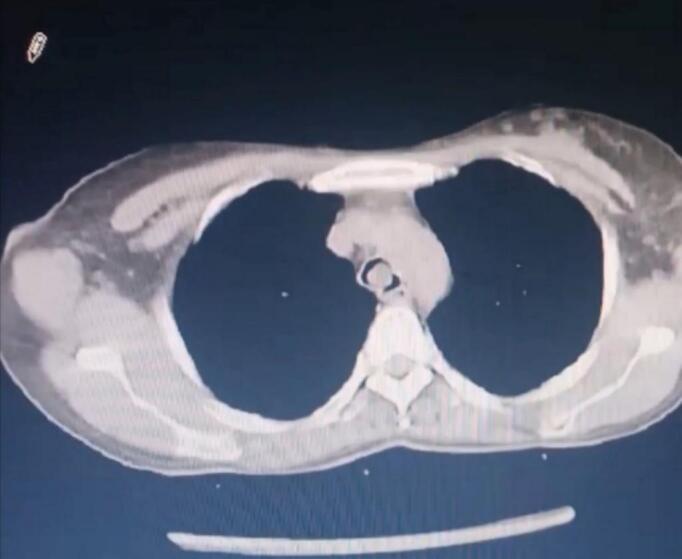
CT scan showing a large lesion obstructing 95 % of the inferior third of the tracheal lumen without extratracheal component.
